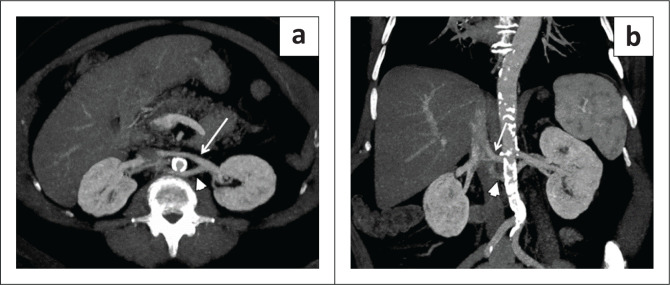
A 61-year-old man who underwent preoperative imaging of the chest, abdomen and pelvis with contrast enhanced CT for aortic valve replacement. (a) Axial CT image demonstrated two left renal veins, one crossing anterior to the aorta (arrow) and the second (arrowhead) coursing posterior to the aorta. (b) Coronal CT multiplanar reconstruction image demonstrated two left renal veins with an inferior location of the retroaortic renal vein (arrowhead).
